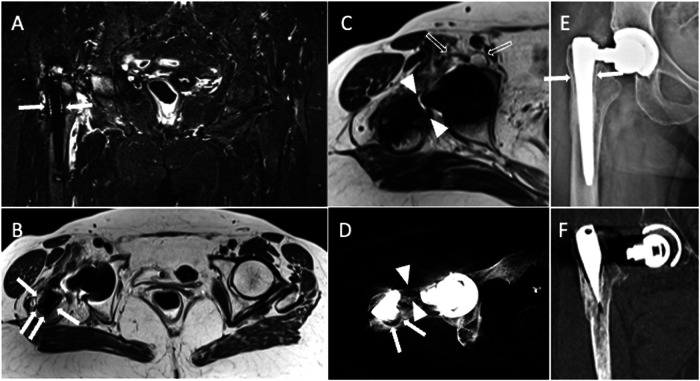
A 77-year-old female with right THA periprosthetic bone resorption and implant rupture. Coronal STIR (A), axial T2-weighted (B), axial CT (D), and AP X-ray view (E) images show fibrous membrane and periprosthetic femoral bone resorption (arrows). Axial T2-weighted (C) and axial CT (D) images show implant neck disruption (arrowheads). Also note ileo-psoas bursitis (void arrows). AP X-ray view (E) and coronal CT (F) image show supraelevation of the femoral stem
